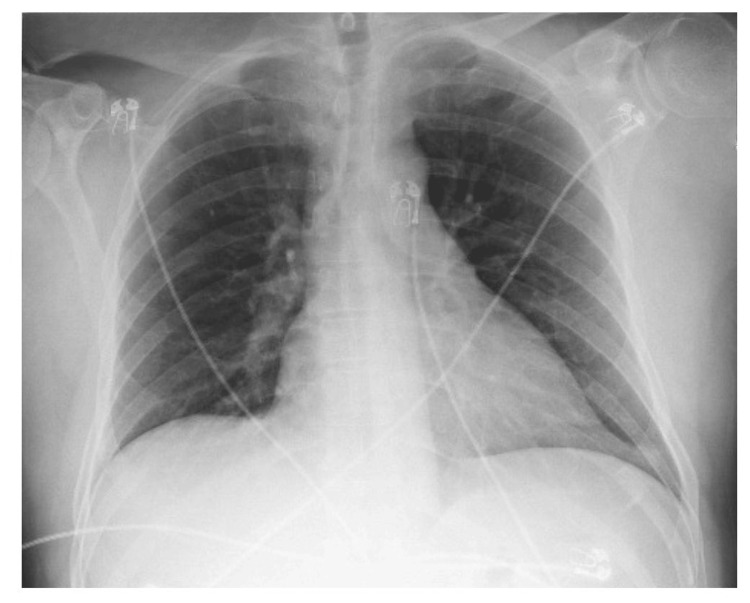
Chest Radiograph Posteroanterior chest radiograph demonstrates a normal cardiomediastinal silhouette. Pulmonary vasculature is normal in caliber and distribution. The lungs are clear bilaterally, with no evidence of consolidation, infiltrates, or masses. No pleural effusions are identified. The costophrenic angles are clear. No pneumothorax is present. Osseous structures are unremarkable.
